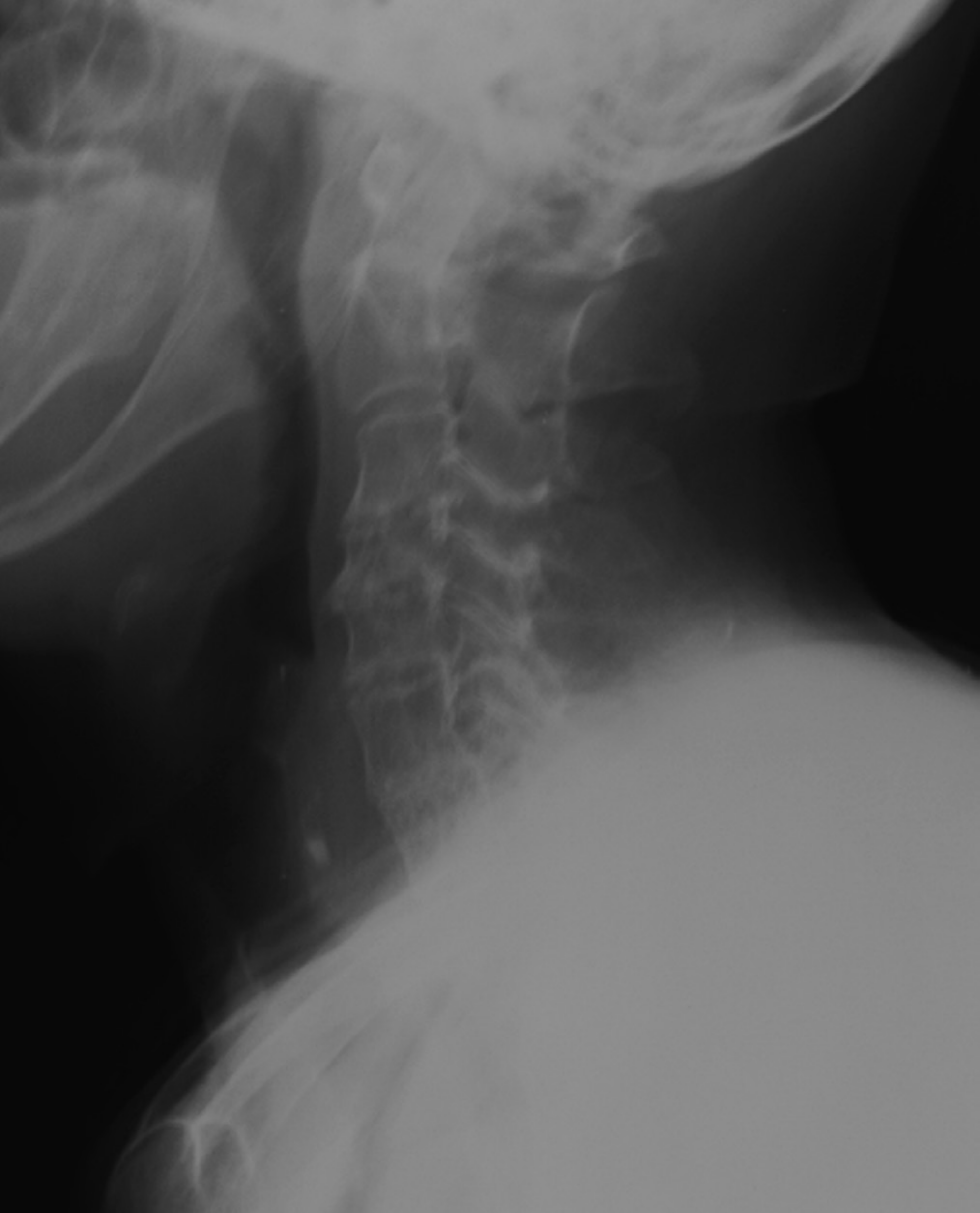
Radiograph of the lateral cervical spine taken during the initial evaluations.
